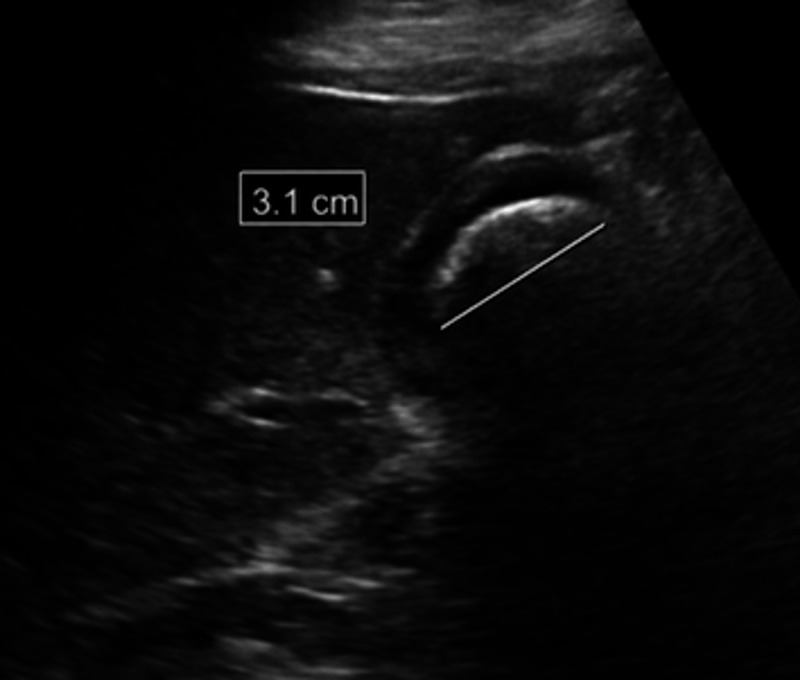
Gallbladder ultrasound showing a large gallstone (3.1 cm) filling the lumen of the gallbladder
